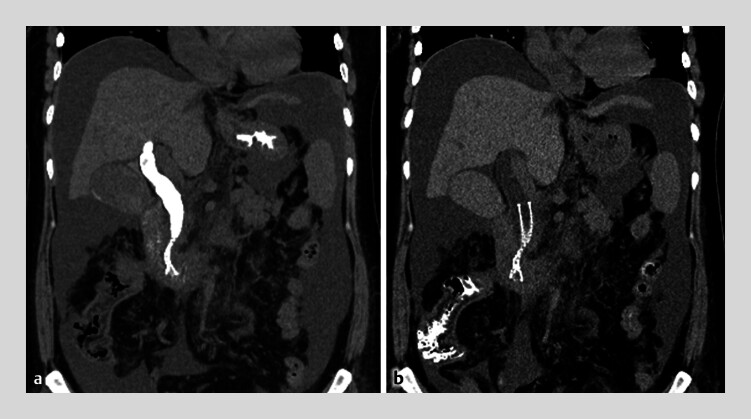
Postoperative computed tomography scan showing:
